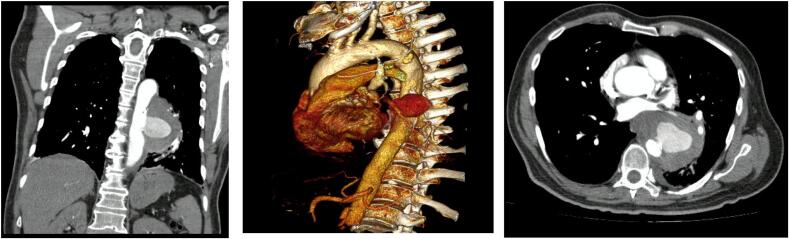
CT angiography with penetrating descending thoracic aortic ulcer contained between 4 and 5th Ishimaru zones.
